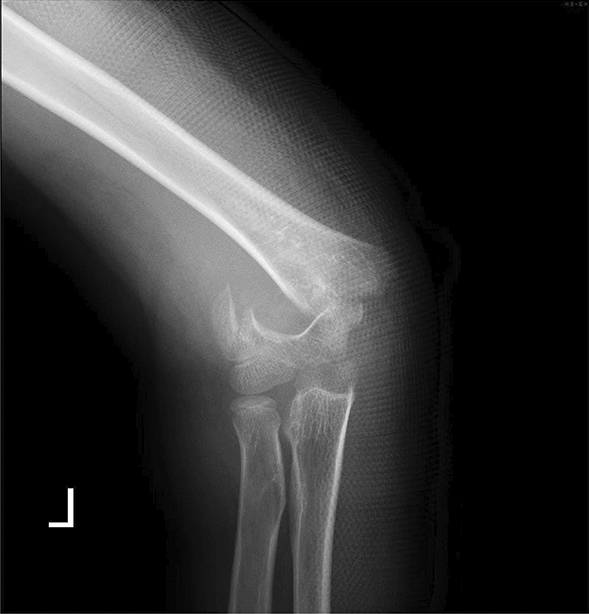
Post-injury X-ray in the anterior-posterior view shows a fracture at the humeral condyle, with significant
